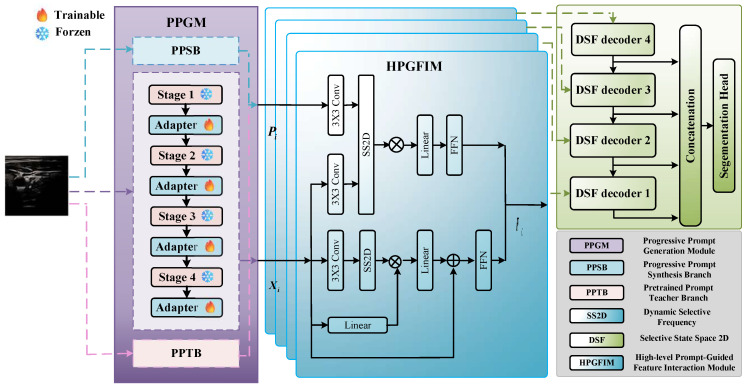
Overall architecture of the proposed RPFeaNet for medical ultrasound image segmentation. It consists of three core components: (1) The progressive prompt generation module (PPGM) with frozen backbone stages and trainable adapters, which generates multi-level spatial prior prompts $P_{i}$ from the input image. (2) The high-level prompt-guided feature interaction module (HPGFIM), which fuses the backbone feature $X_{i}$ with the prompts $P_{i}$ via Selective State Space 2D (SS2D) blocks to enhance target-aware feature representation. (3) The dynamic selective-frequency (DSE) decoder stack, which refines the fused features in the frequency domain and produces the final segmentation map through a concatenation head.
